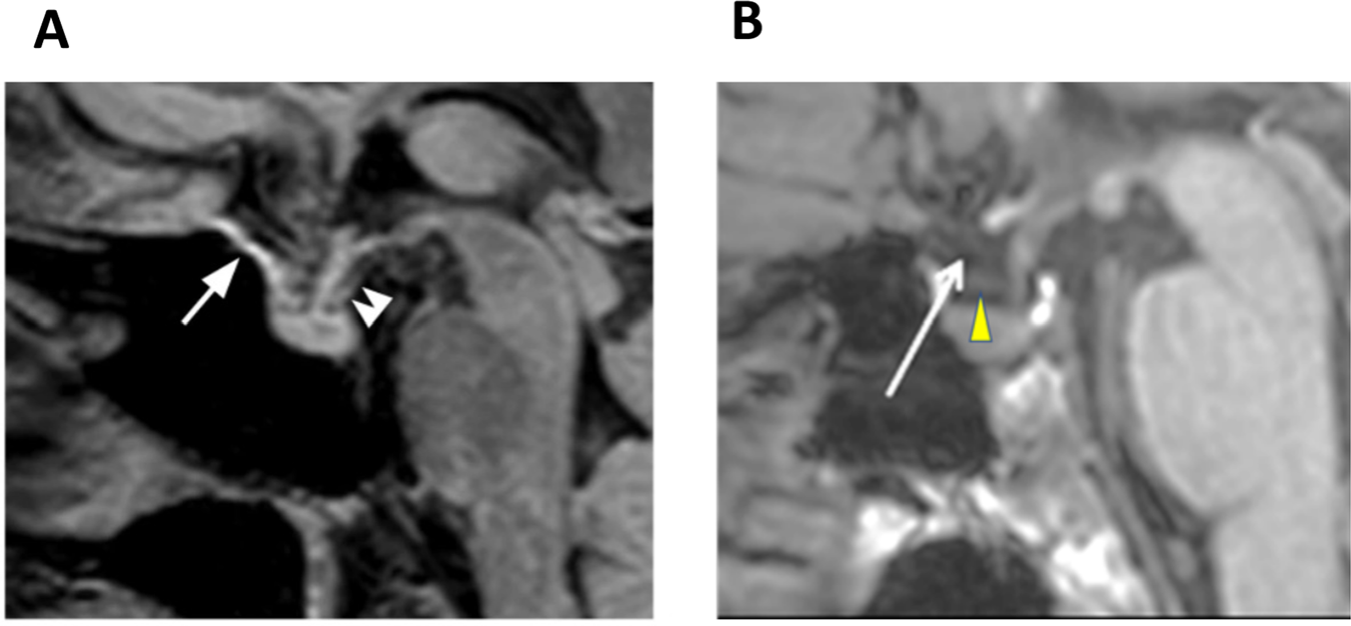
Visualization of the diaphragma sella. A Sagittal FLAIR images show the putative ventral dural lymphatic elements in the diaphragma sella (double arrowhead; (adapted from Figure 4c of Albayram et al., *Nat. Commun*. 13, 203 (2022)^1^). B Moreover, we can identify a subtle linear pattern, most likely the diaphragma sella (yellow arrowhead) in Ringstad et al. Figure 1c^2^, just under their arrow, as presented below (with contrast adjustment); (adapted from Fig. 1c at Rinstad et al., *Nat. Commun*. submitted).
